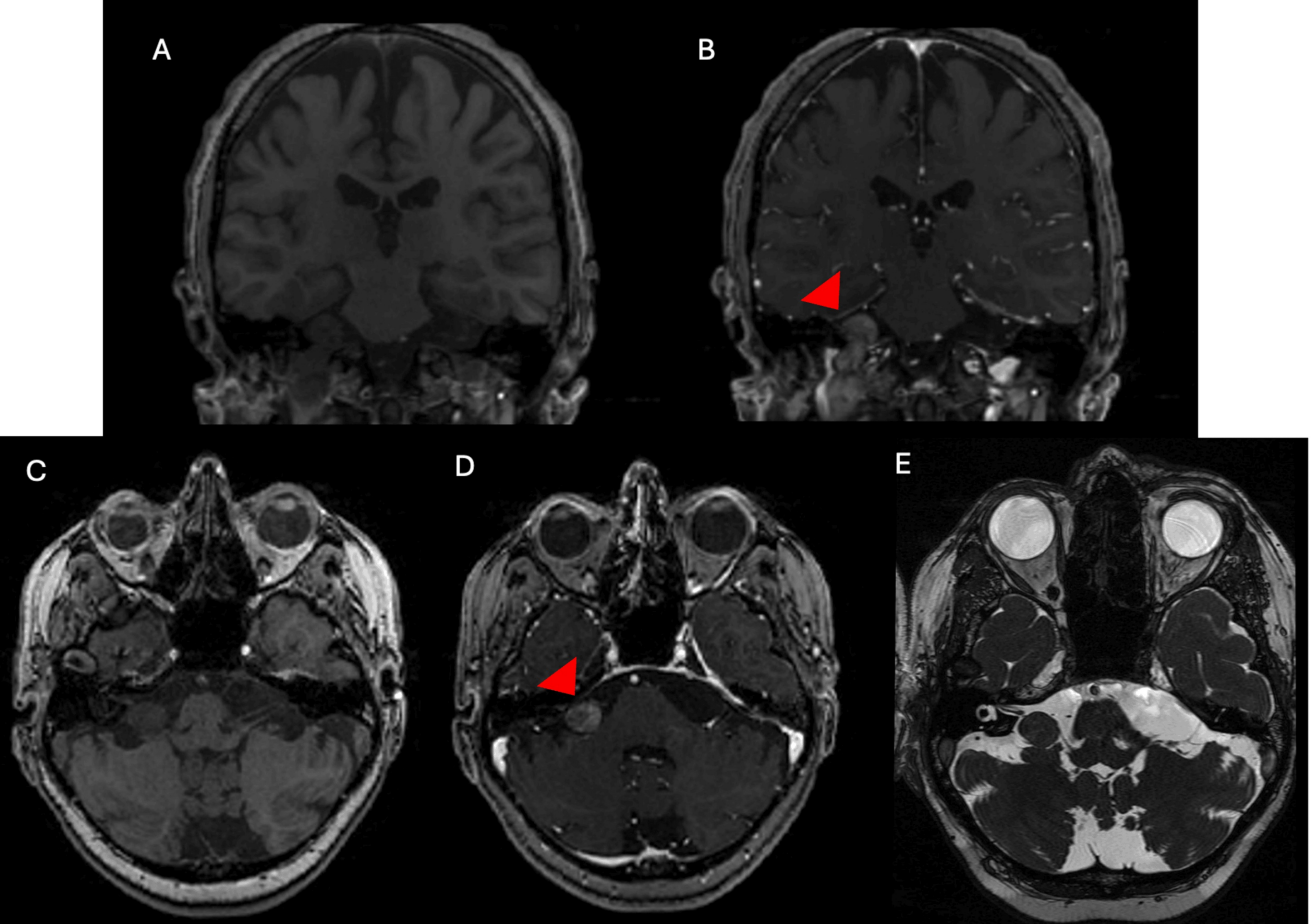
MRI characteristics of the jugular foramen schwannoma prior to radiation therapy. A dumbbell-shaped lesion centered at the jugular foramen is shown (A, B) (red arrowhead), with extension into the cerebellopontine angle cistern (C-E). The lesion demonstrates heterogeneous enhancement following gadolinium administration (B, D) (red arrowhead) and exhibits mixed signal intensity on fast imaging employing steady-state acquisition (FIESTA) sequences (E).
